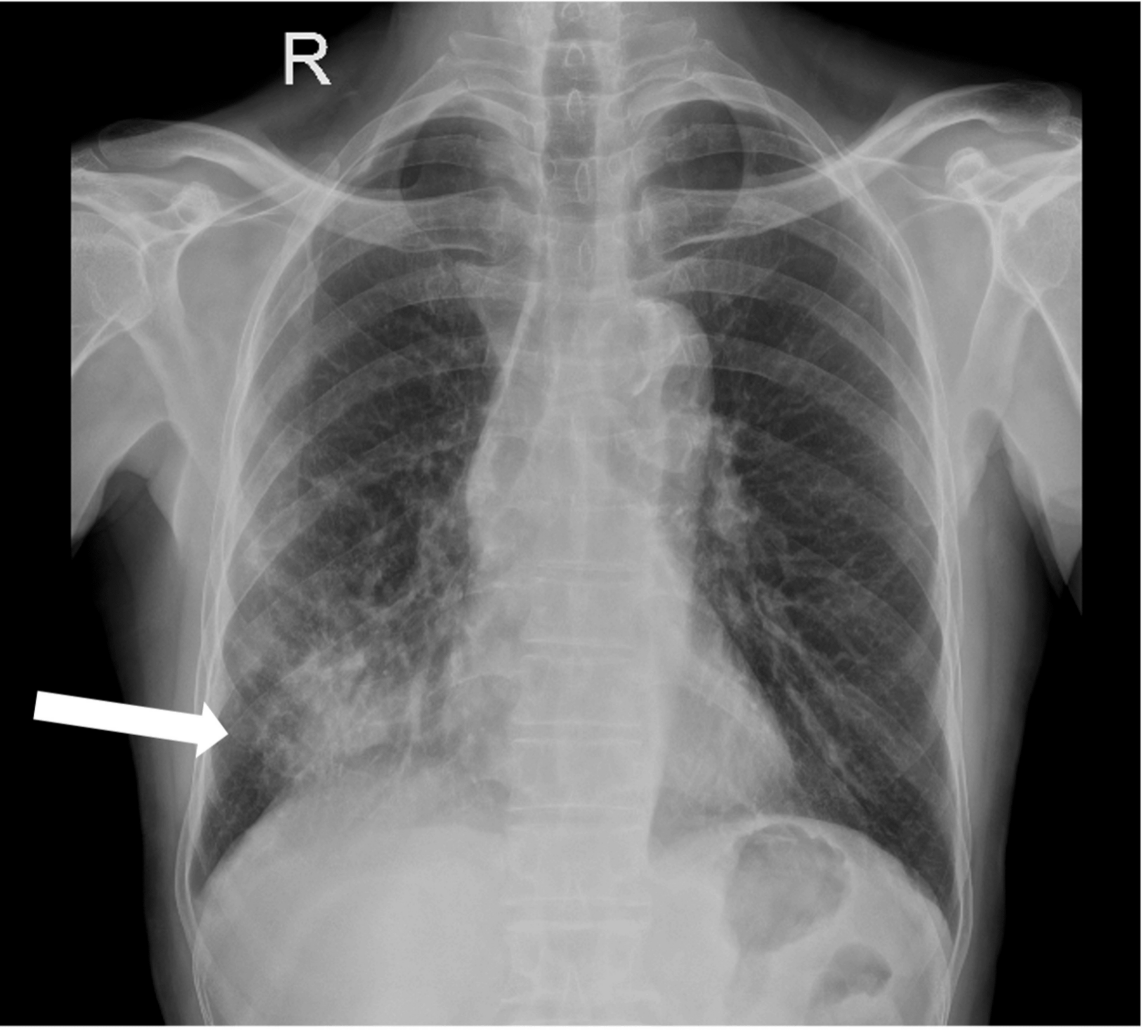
X-ray showing right lower zone heterogeneous opacities
